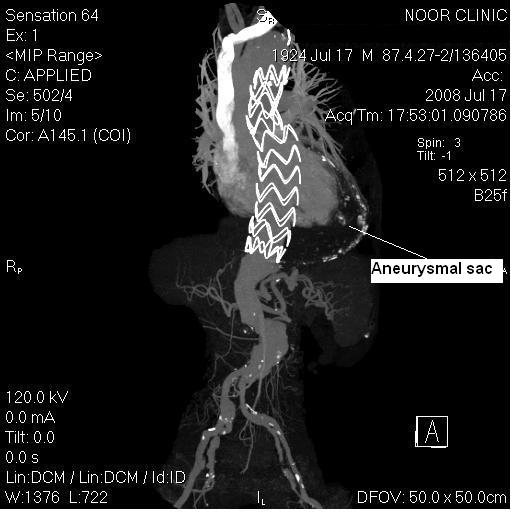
Follow-up computed tomography angiography showing a total exclusion of the aneurysm by the stent graft.
